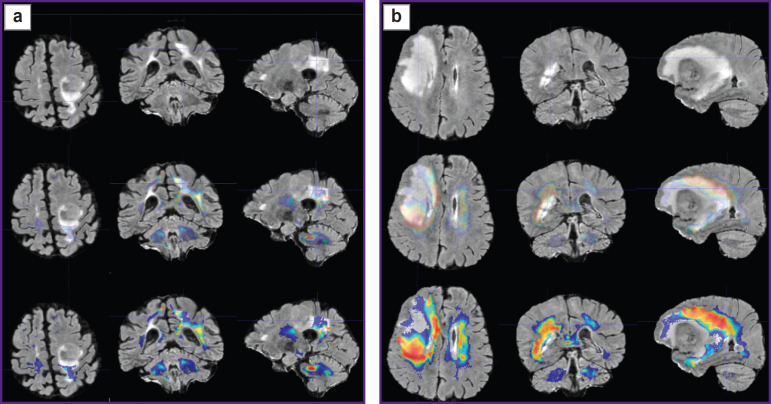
Plotting the maps of value gradients of mean kurtosis (МК) in patient with glioblastoma (а) and in patient with metastasis (b) The upper row — T2-FLAIR images in three projections; middle row — comparison of T2-FLAIR with MK parametric map (semitransparent for better contour visualization of the changed MR signal by T2-FLAIR); lower row — comparison of T2- FLAIR with MK parametric map (in full color, where red and yellow tones show the zone of greatest parameter values)
